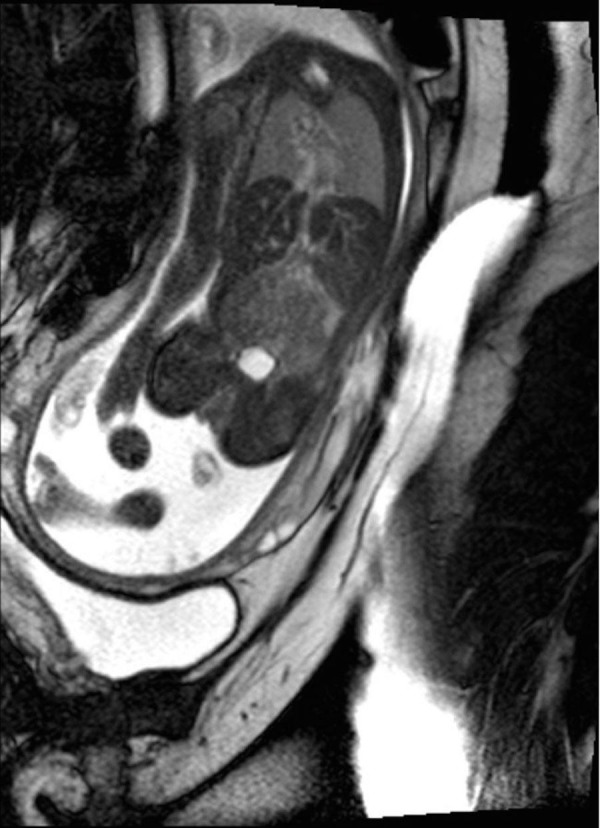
Bronchial isomerism A coronal T1-weighted image demonstrating bronchial isomerism with bronchial angles consistent with right isomerism. There is also a midline liver noted in this slice.
